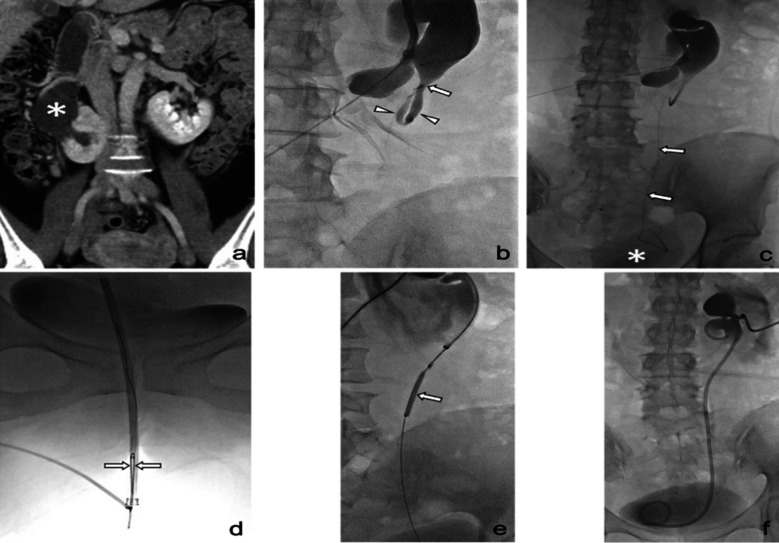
A 44-year-old woman (Patient #2) with underlying horseshoe kidney and fibromatosis underwent Double-J stent placement with the through-and-through wire technique. (a) Coronal contrast-enhanced computed tomography showed right hydronephrosis (asterisk) involving right ureteropelvic junction (UPJ) obstruction. (b) An antegrade pyelogram with contrast medium injected through a 5F catheter showed stenosis of the UPJ (arrow) and a tortuous proximal ureter (arrowheads). (c) After failed stricture negotiation using a 0.035-inch guidewire, there was successful passage of a 0.018-inch microguidewire (arrows) inserted through a 2F microcatheter, through the stricture, and into the urinary bladder (asterisk). (d) The tip of the microguidewire was captured by a snare (arrows) through a 9F sheath in the urinary bladder and withdrawn out of the urethra. (e) After the microguidewire was substituted with a 0.035-inch guidewire (not shown), serial 4-, 6-, and 8-mm balloon dilation (arrow) was performed at the stricture. (f) An 8F Double-J stent was successfully placed.
